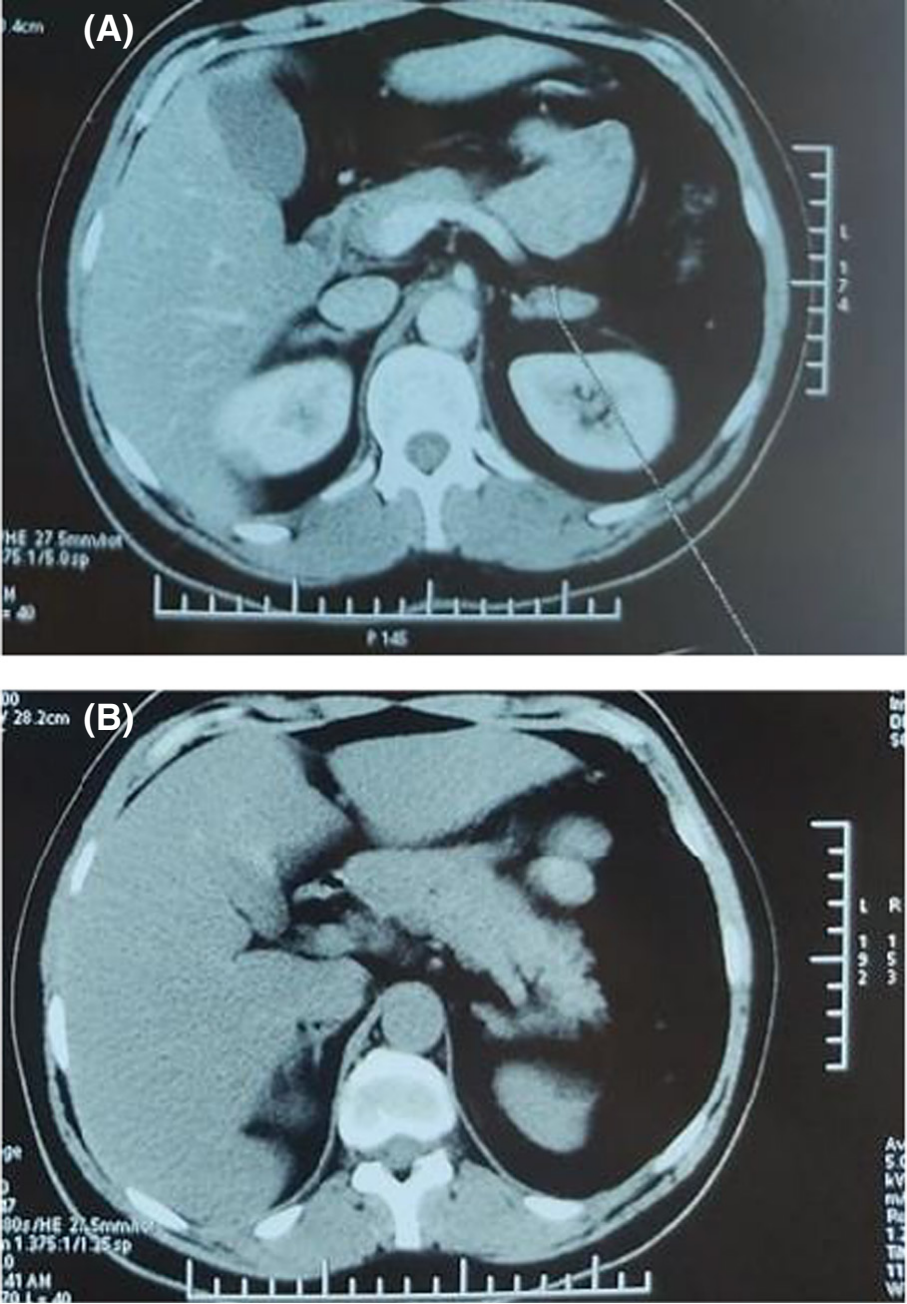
(A, B) CT scan of the abdomen demonstrating two adenomas in the left and right adrenal glands, measuring 30 × 17 mm and 15 × 9 mm, respectively.
